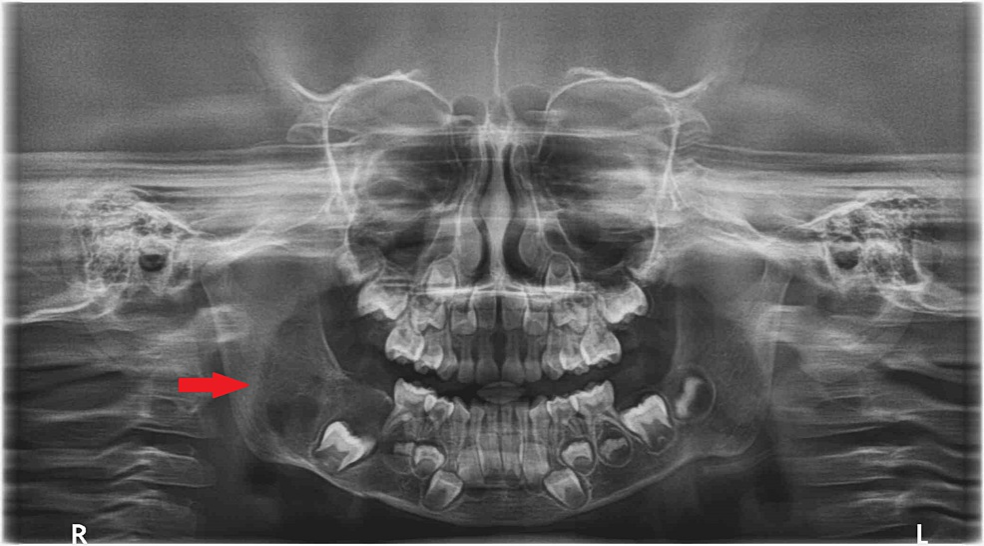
A panoramic radiograph taken during the one-year follow-up The red arrow shows persistent bone consolidation around the right mandibular angle.
